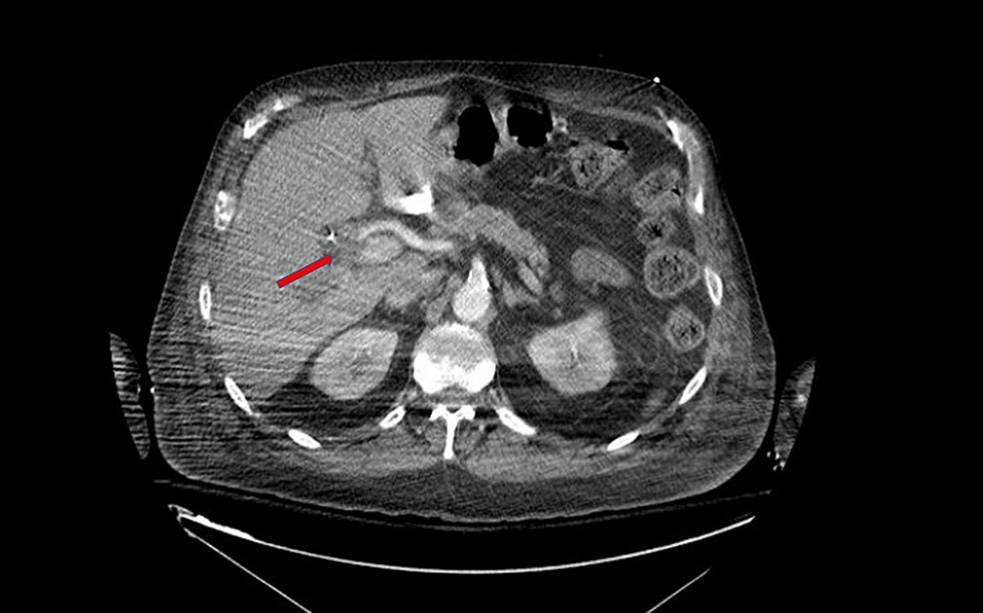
Axial view of clipped right hepatic artery seen on CTA abdomen This figure demonstrates the common hepatic artery branching into the right and left hepatic arteries. A red arrow is pointing toward the right hepatic artery, which was ligated and clipped during the patient's initial surgery. computed tomography angiography (CTA)
